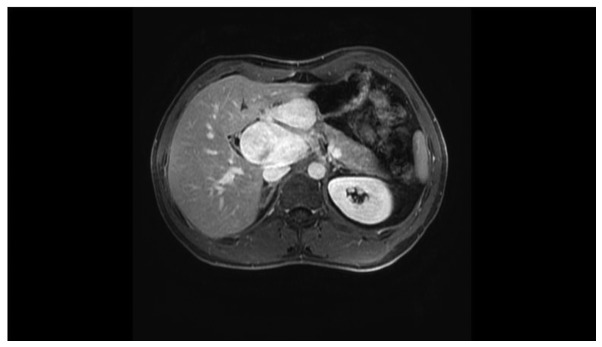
Axial T2-weighted fat saturation sequences show a marked hyperintense pancreatic head mass that abuts the hepatic artery.
